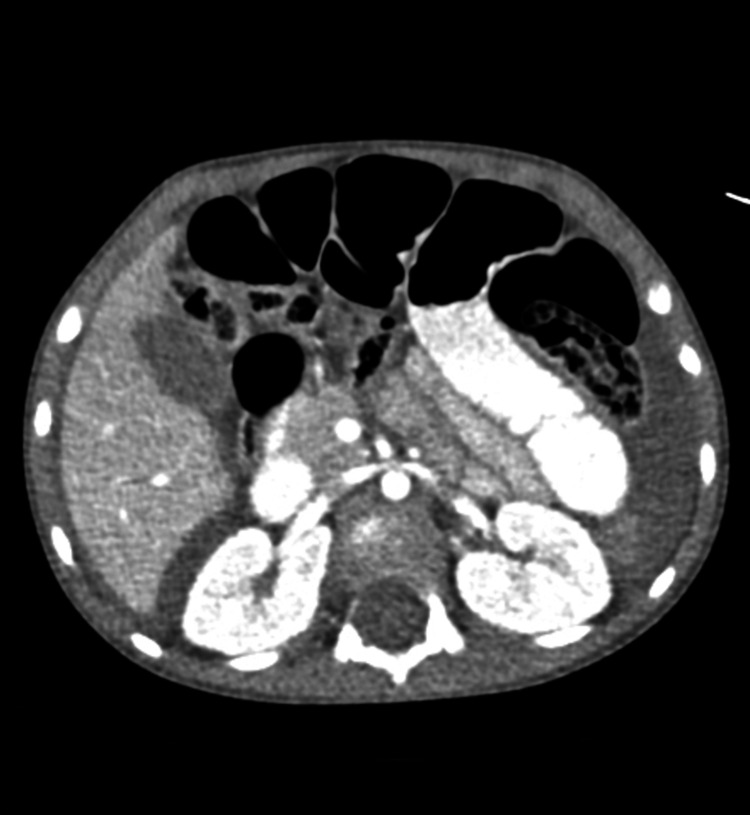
CT scan of the abdomen and pelvis showing no visualization of the appendix, dilated small bowel loops, and surrounding inflammatory changes distal to the area of obstruction. CT: computed tomography
